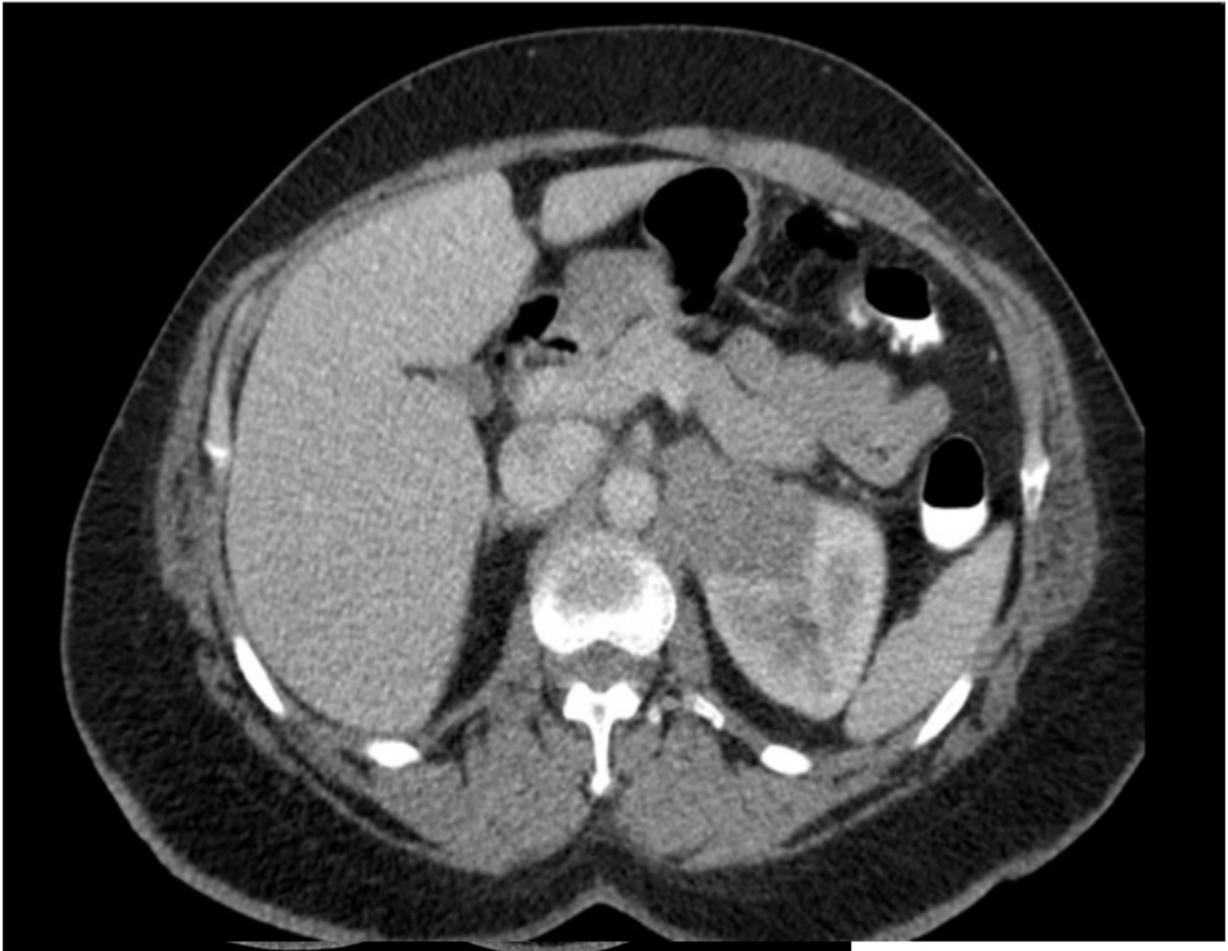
Contrast-enhanced CT scan of the abdomen showing left kidney mass at initial presentation.
